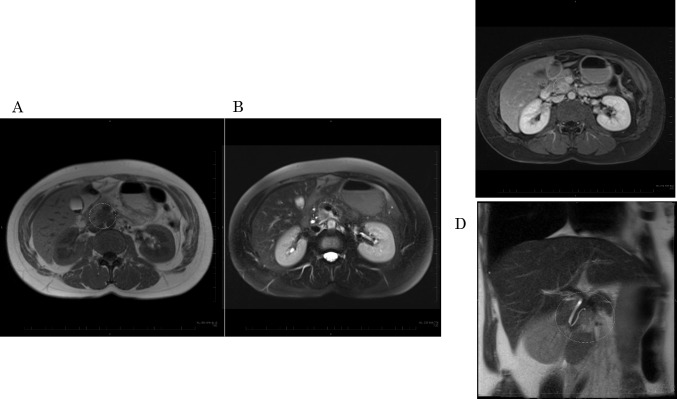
MRI. A: T1WI, B: T2WI, C: enhanced MRI, D: MRCP. C: Low-intensity in the early phase. High periphery intensity and iso-central intensity in the late phase. D: Mass is adjacent to the pancreatic duct but there is no stenosis
